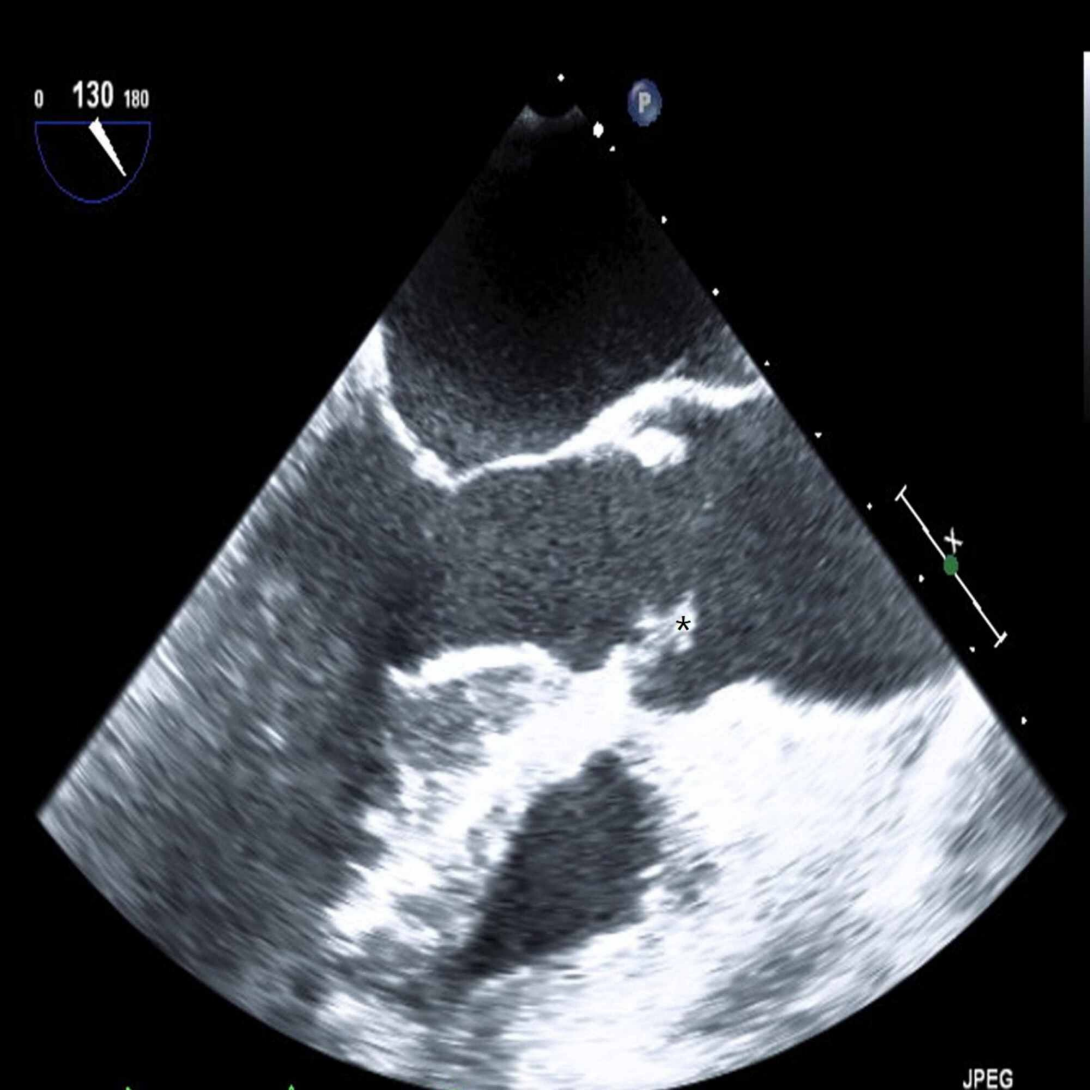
Transesophageal echocardiography with aortic long-axis view showing echo-density in aortic valve which suggestive of vegetation (black asterisk)
